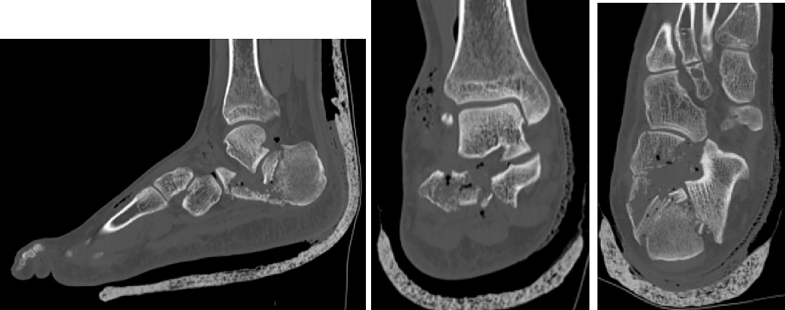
Sagittal, Coronal and Axial CT-scan after initial splinting in ER.
